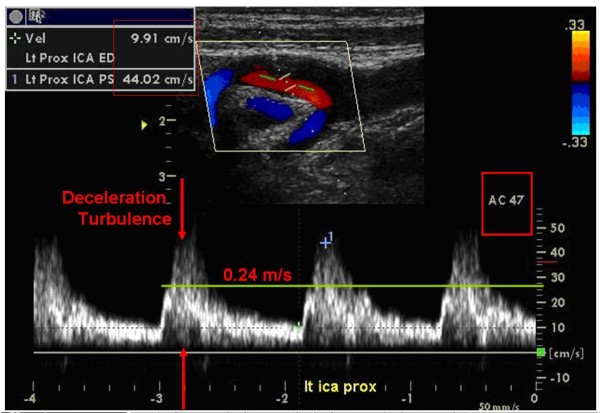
End Acceleration Velocity Followed by Deceleration Turbulence. Cursor 1 was placed by the examiner at the traditional location for peak systolic velocity. The Green line was added by the UWURC Reader at the End Acceleration Velocity; the red annotation was added by the UWURC Reviewer. The Doppler Angle is 47 degrees
